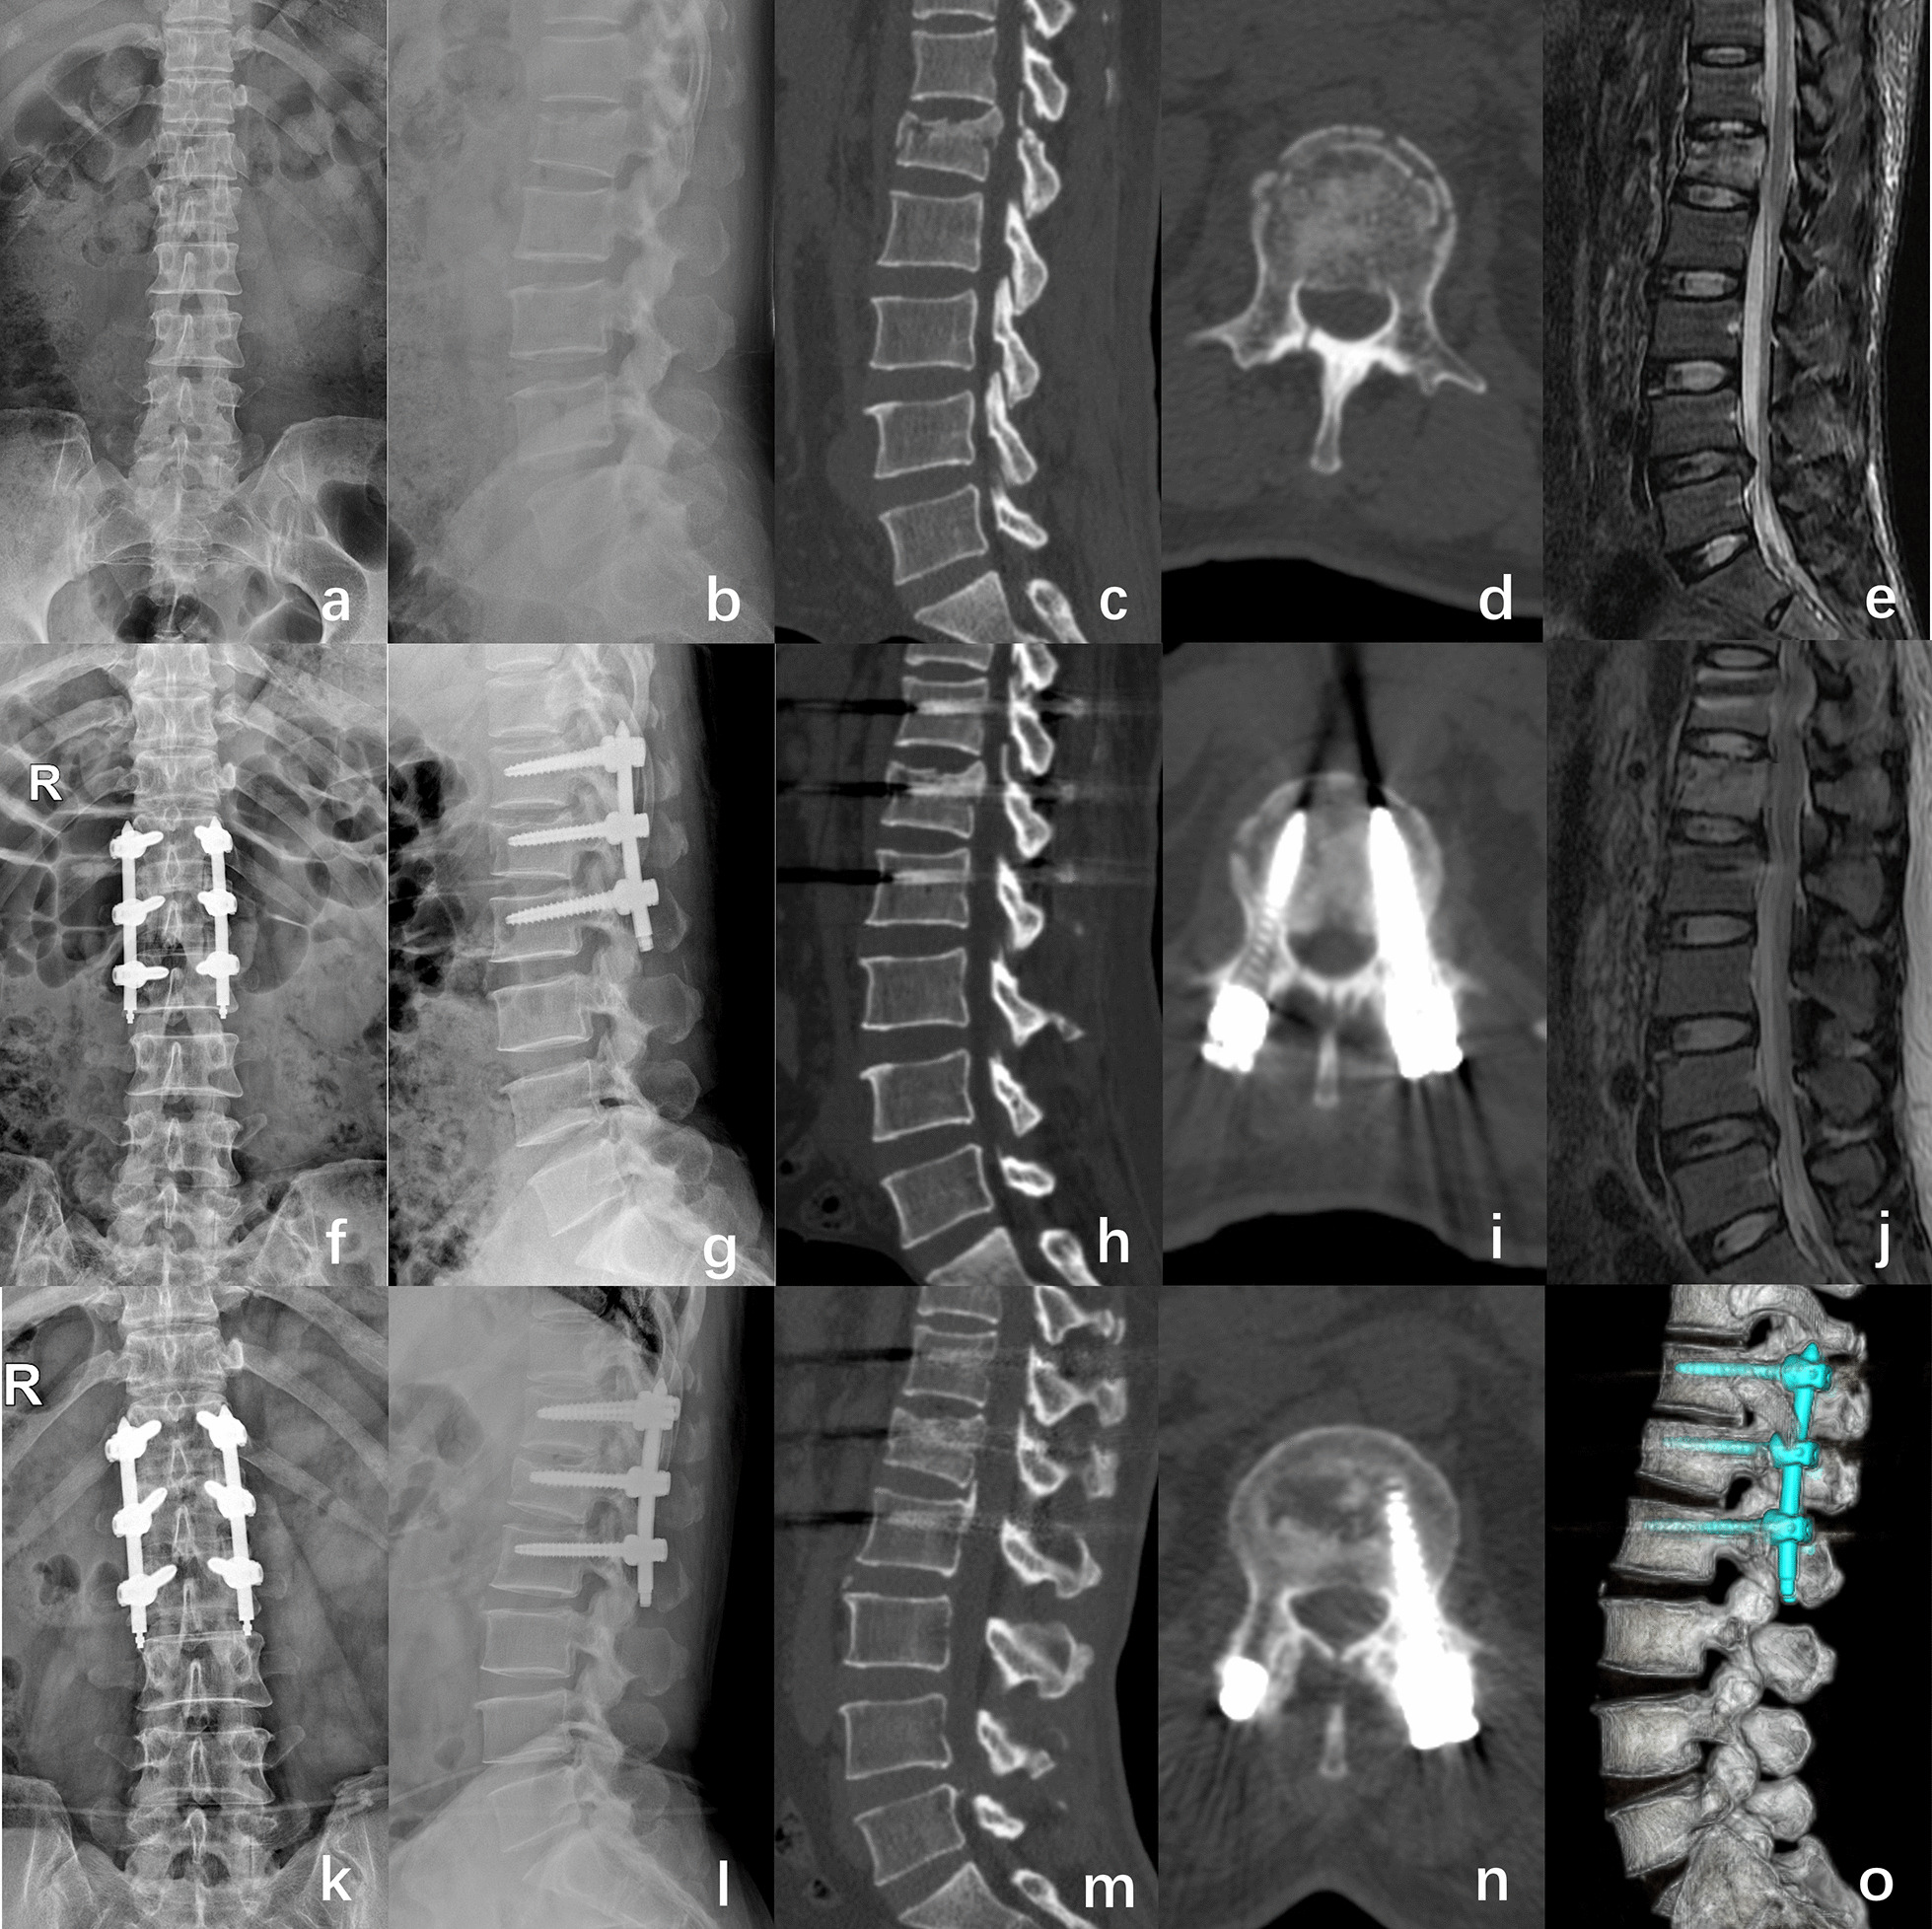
A 46-year-old female patient with L1 burst fracture was treated with MSs fixation. a–d Preoperative lumbar radiographs (a, b) and CT (c, d) demonstrated a L1 AO type A3 fracture with spinal canal encroachment. e MRI showed a fresh fracture of the L1 vertebrae. f–i One-week postoperative radiographs (f–g) and CT (h–i) showed satisfactory injury vertebral height restoration and kyphosis correction. j One-week postoperative MRI show the spinal canal encroachment decreased. k–l Radiography at the last follow-up at 12 months after surgery showed that the internal fixation systems were stable with good positioning and good morphology. m–o And CT showed that spinal canal encroachment was remarkably relieve
